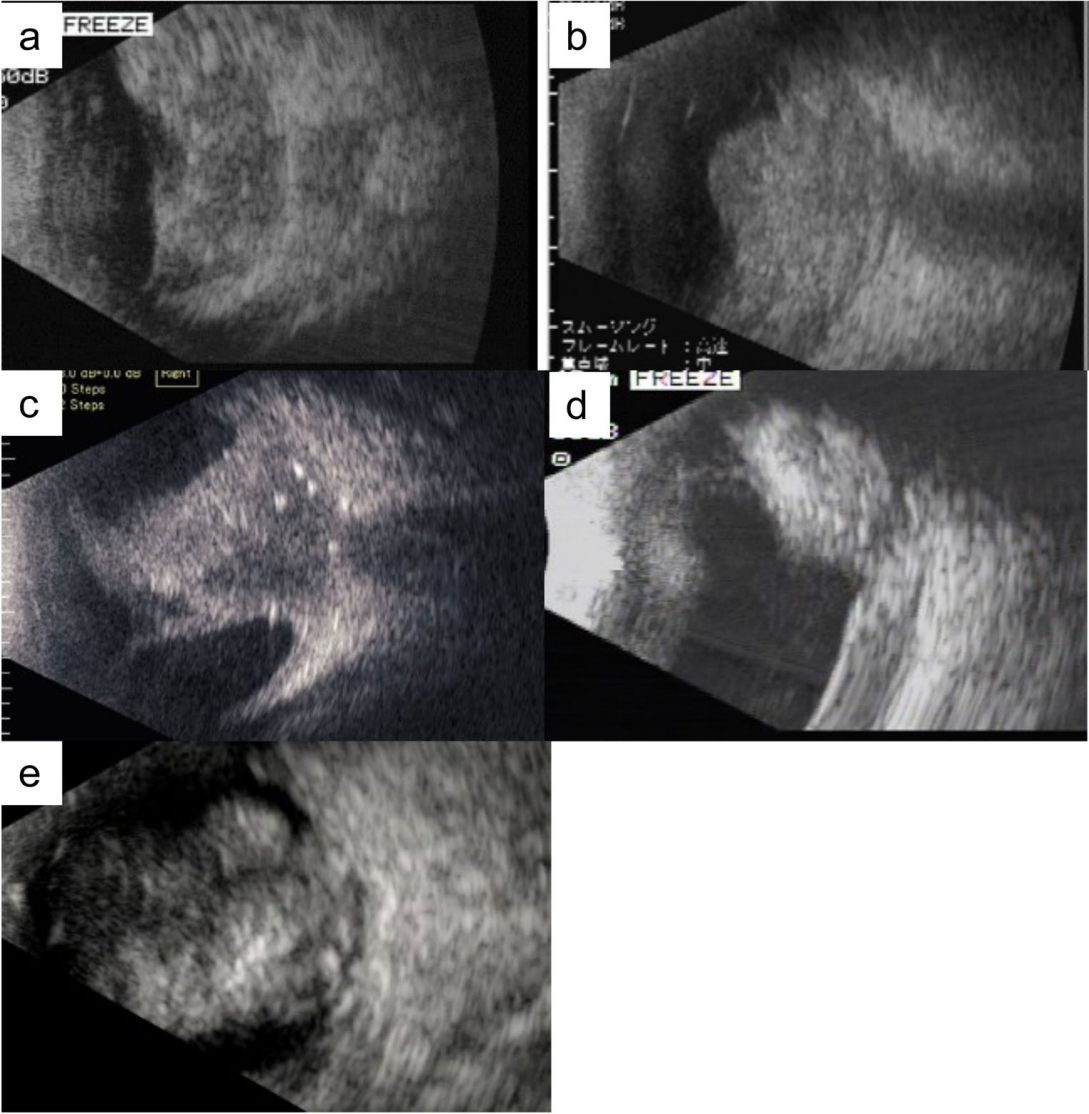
B-mode ultrasonograms of cases 1–5 (a-e). A solid tumor mass with highly reflective foci is seen in each case, but no obvious invasion of the optic nerve (ON) is detected in any case
